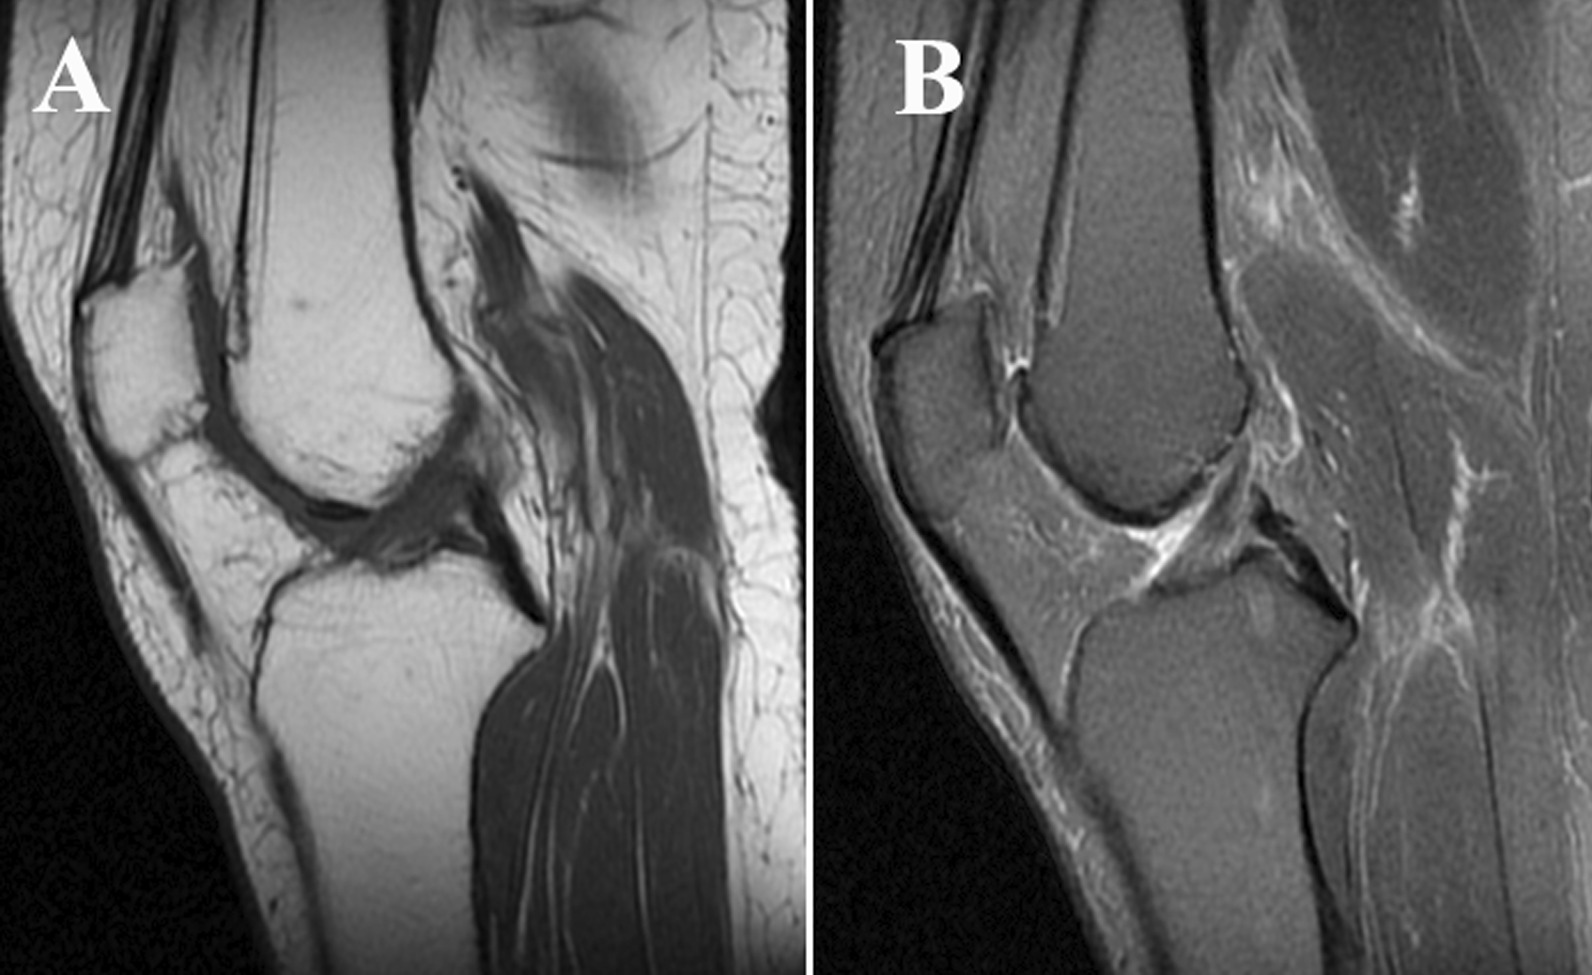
Postoperative MRI showing clear knee joint space and no synovial thickening. A. T1WI, B. T2WI
